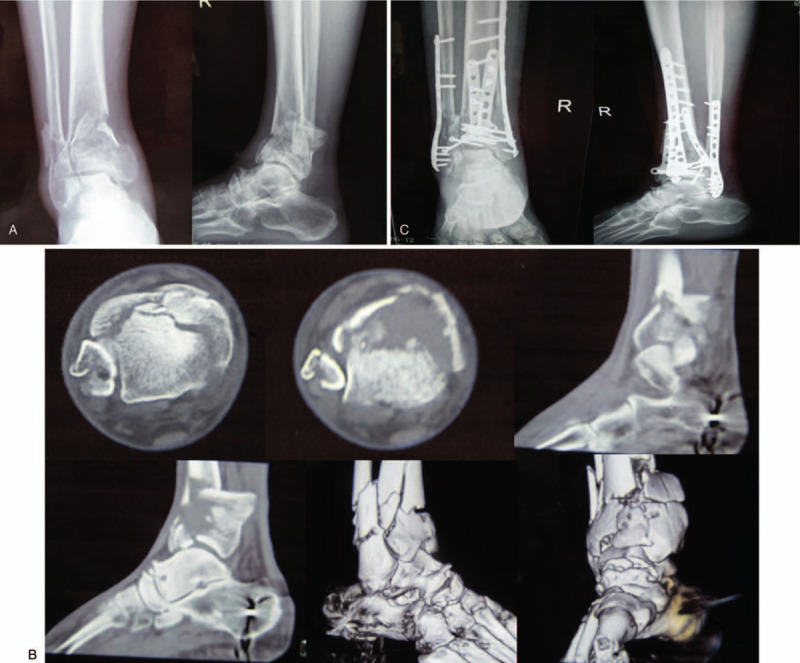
A. Preoperative X-rays. B. Preoperative CT scans and 3D reconstruction. C. Postoperative X-rays.
